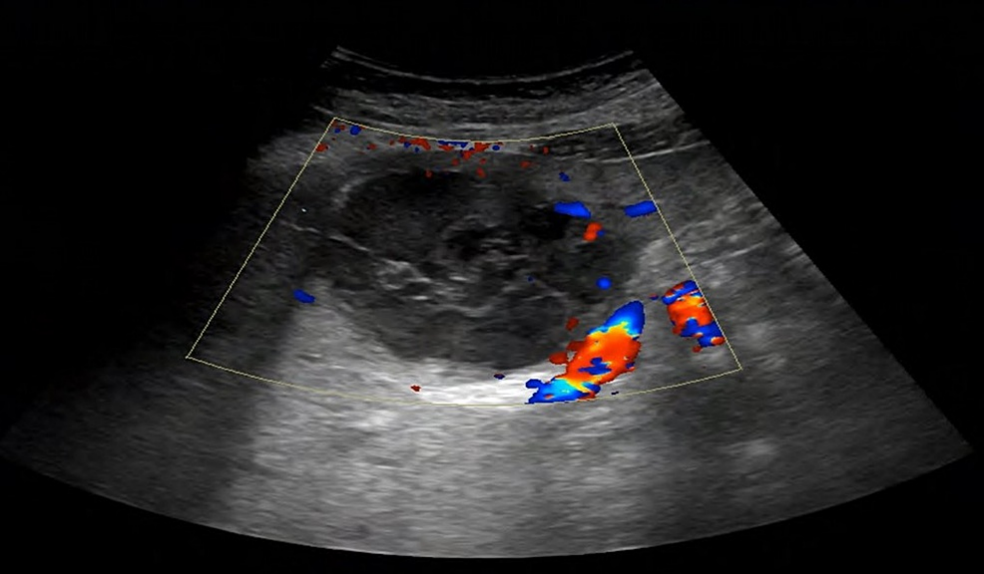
Liver ultrasound showing a complex cystic mass lesion with vascularity in the wall and the septae
